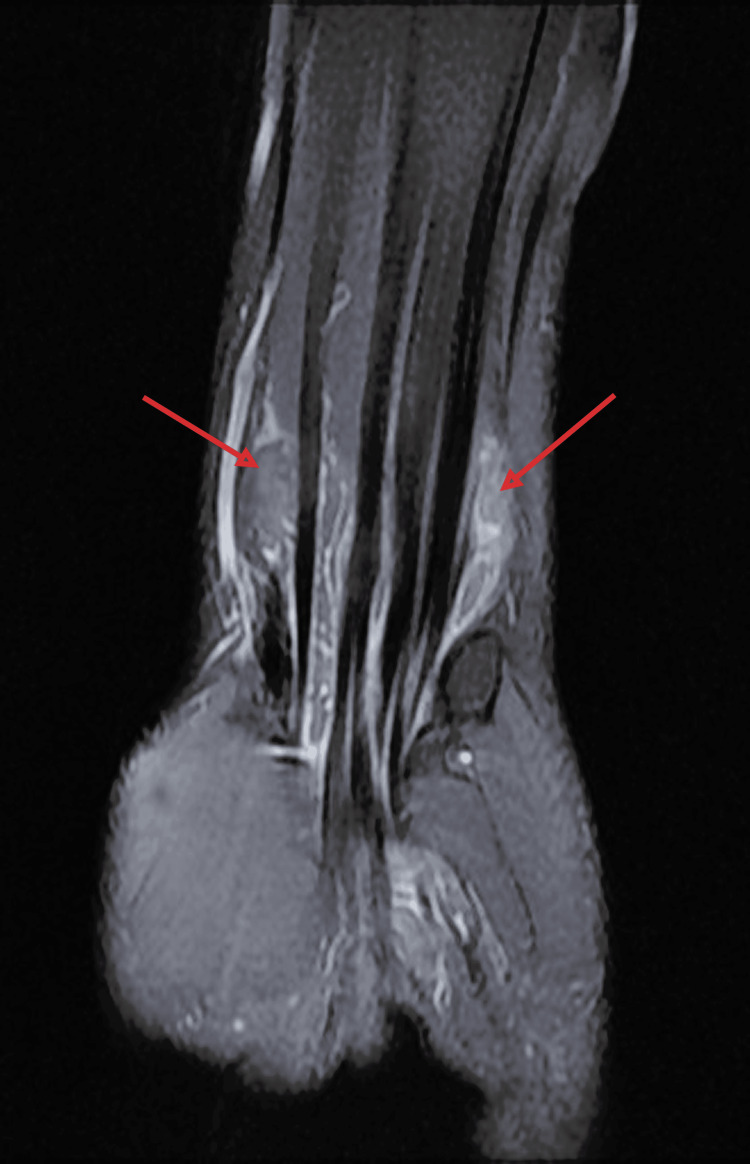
Proton density fat-saturated coronal MRI of the wrist The image is showing fluid collections along flexor tendon sheaths, suggestive of tenosynovitis.
